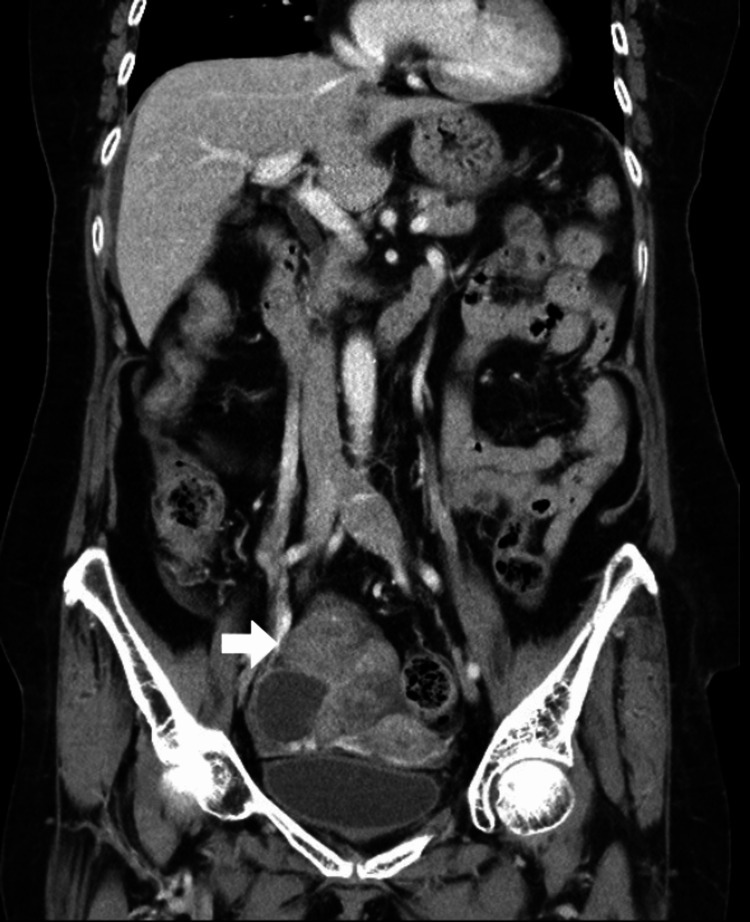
Contrast-enhanced computed tomography of the pelvis A large intraperitoneal lobulated, isodense and heterogeneously enhancing mass (70 x 68 x 58 mm), shown with an arrow, with internal hypoenhancing and cystic components was demonstrated at the right superior aspect of the uterus, with compression on the uterus and superior wall of the urinary bladder.
